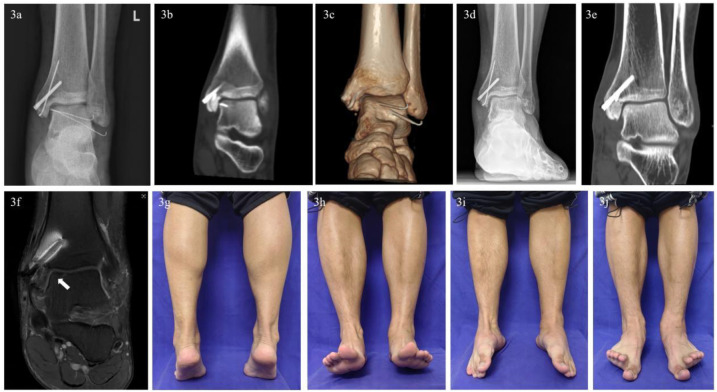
Postoperative follow-up: imaging performed two months postoperatively (a–c) confirmed satisfactory healing of the osteochondral fragment of the talus. At one year postoperatively, a weight-bearing anteroposterior x-ray (d) showed a well-aligned ankle joint. Further CT and MRI scans (e, f) revealed proper healing of the talar cartilage, with resolution of bone marrow edema signals in the talus. Functional evaluation at one year (g–j) demonstrated excellent recovery of ankle joint function.
